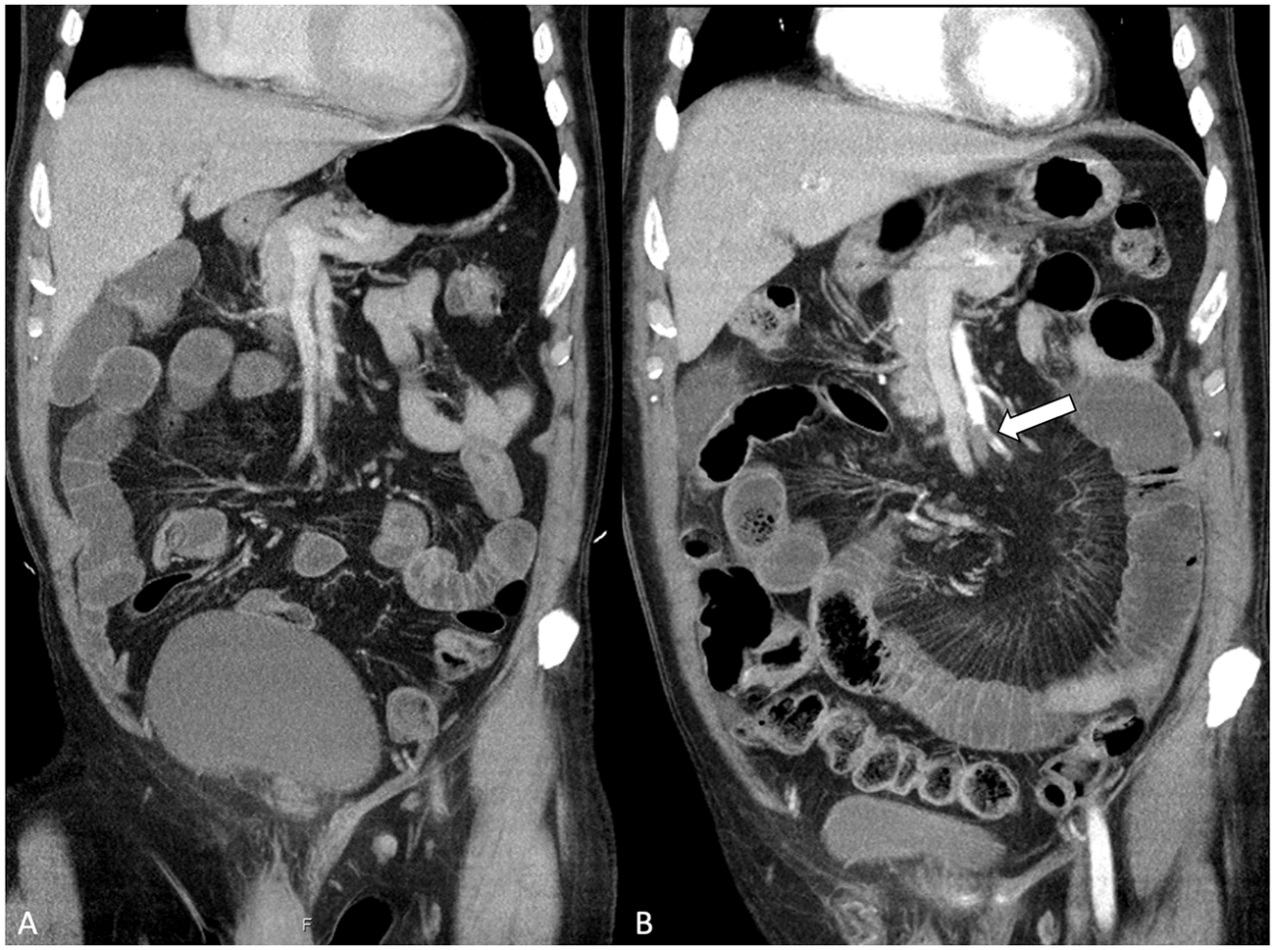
A) The contrast-enhanced computed tomography (CT) of acute abdomen performed in venous phase alone without suspicion of acute mesenteric ischemia (AMI) prior to the imaging. The embolic occlusion of the superior mesenteric artery (SMA) is vaguely visible and was missed in the initial CT examination. B) A second imaging was obtained, this time with suspicion of AMI mentioned in the CT referral. The second CT was performed with split bolus protocol, which consists of contrast enhancement in venous phase and a second bolus in the arterial phase. This time, the SMA embolus is clearly visible (arrow).
